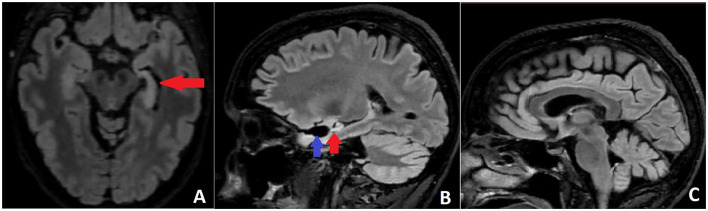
(A) Axial FLAIR MRI with left HS (red arrow). (B) Sagittal FLAIR MRI scan demonstrating the postoperative defect (blue arrow) and the left HS (red arrow). (C) Sagittal FLAIR MRI without obvious signs of cerebellar and brainstem atrophy.
